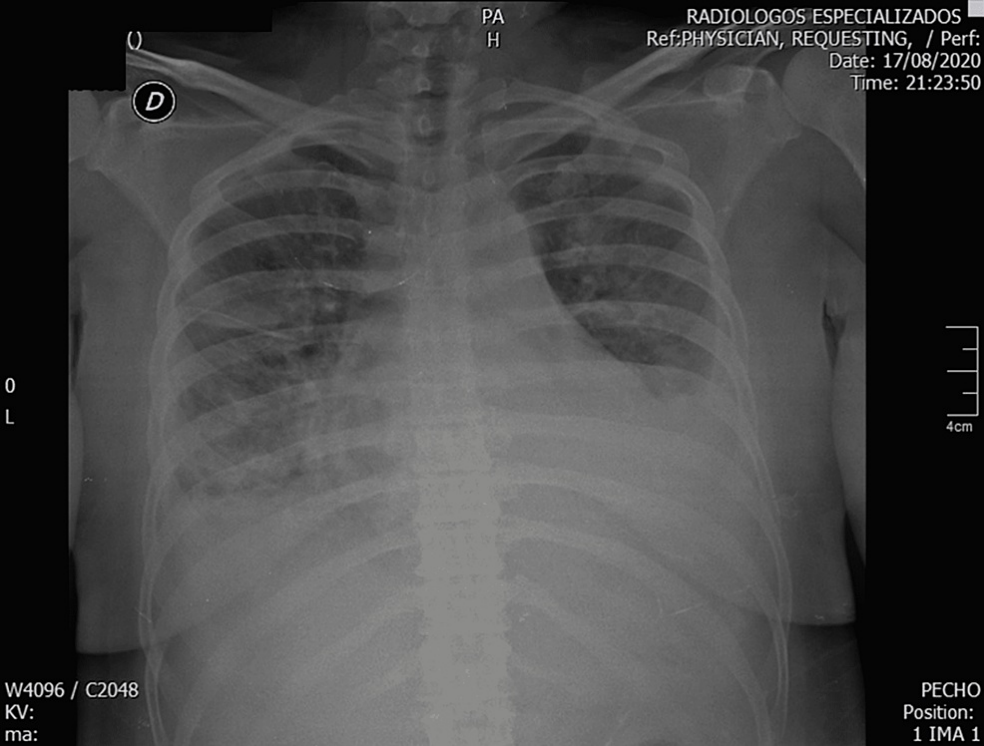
Chest X-ray Cardiac silhouette increased in size, a widening of the upper mediastinum of probable vascular origin is shown, and the costodiaphragmatic angles are blurred by pleural effusion, predominantly on the left side. Multiple diffuse distribution opacities with a tendency to bibasal consolidation are observed in the lung fields.
